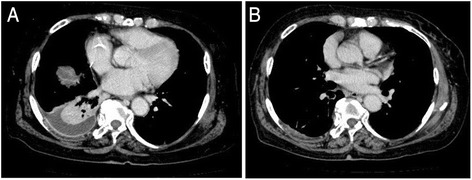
a Two-week postoperative chest-abdominal CT shows an abscess in the right thoracic cavity. b Six-week postoperative CT shows disappearance of the abscess in the right thoracic cavity
